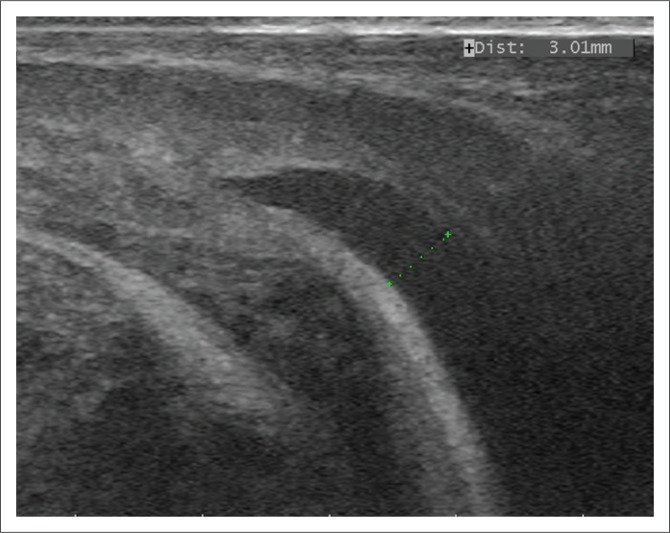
An ultrasonographic evaluation of the distal urethra showing a hyperechoic mass obstruction, 3.75 mm in diameter, proximal to the glans penis.
